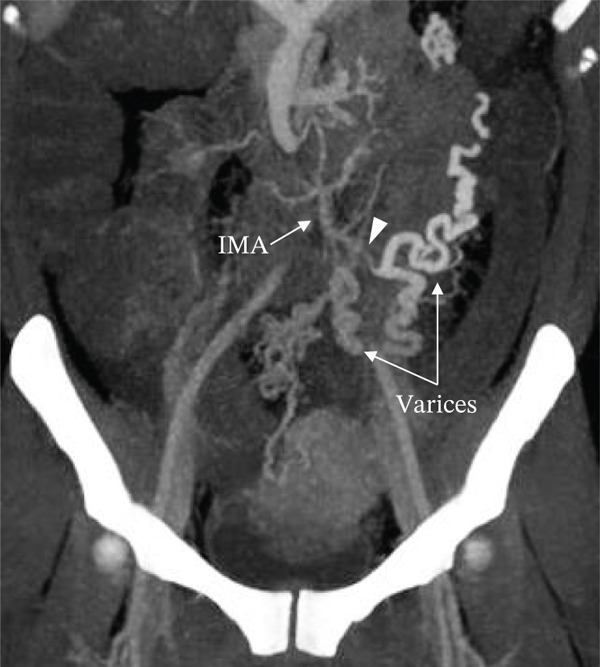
Coronal contrast‐enhanced CT obtained at an outside hospital 2 weeks preprocedure. Between the IMA and dilated mesenteric varices, a possible arteriovenous communication can be seen on retrospective review (arrowhead).
